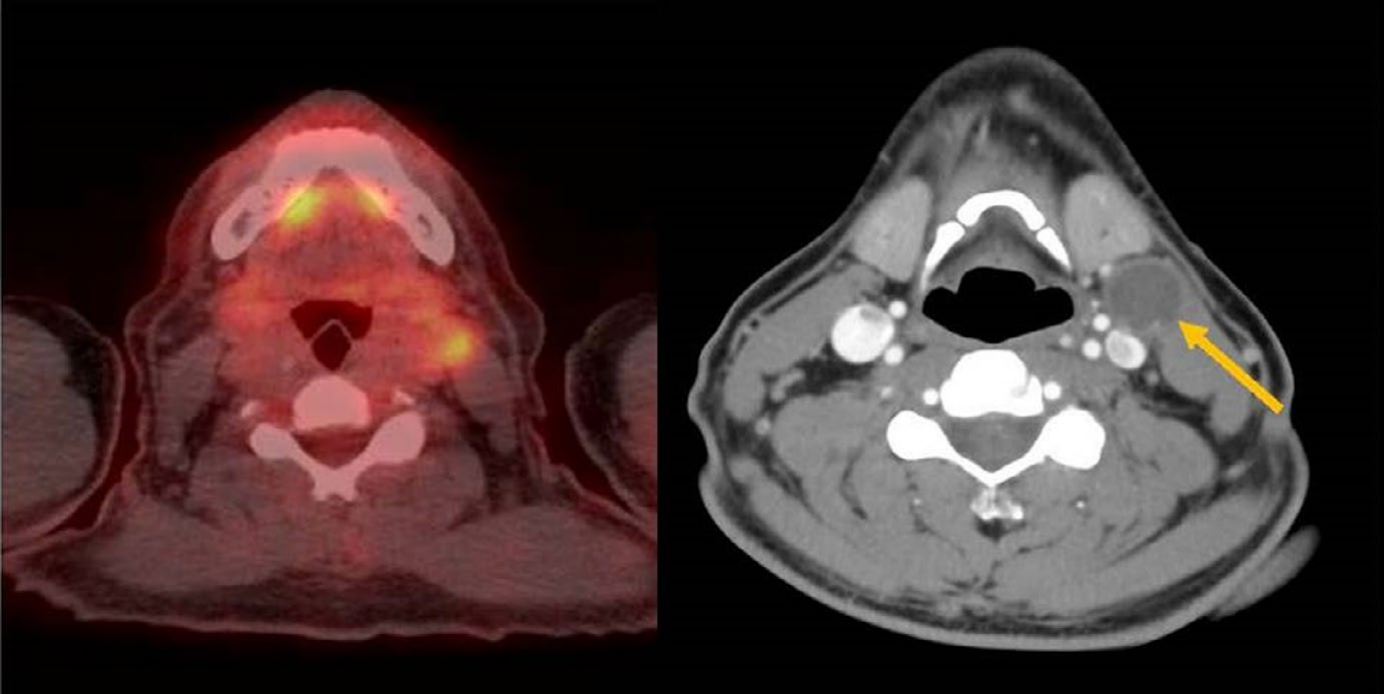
PET/CT scan showing mild increased uptake in the 3.2‐cm cervical node
